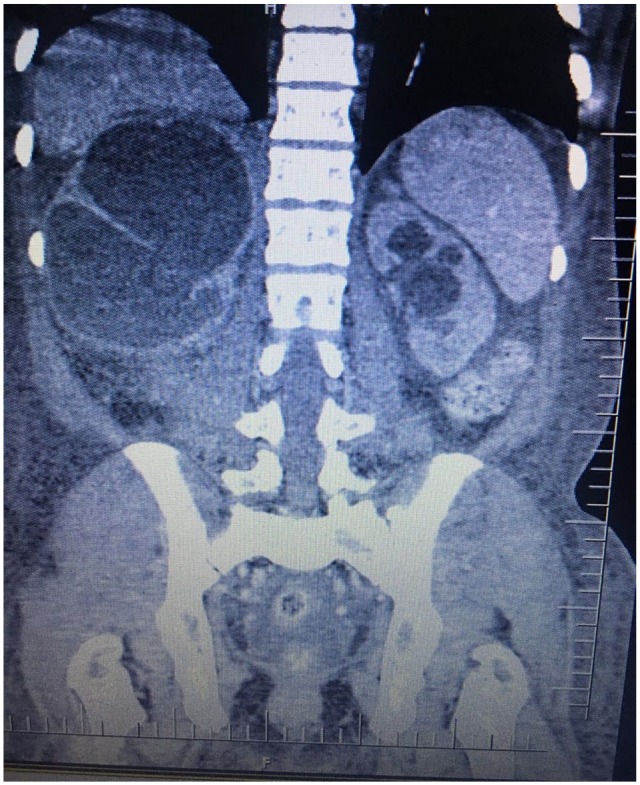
Computed tomography scan of the abdomen (coronal reformatted view) showing
